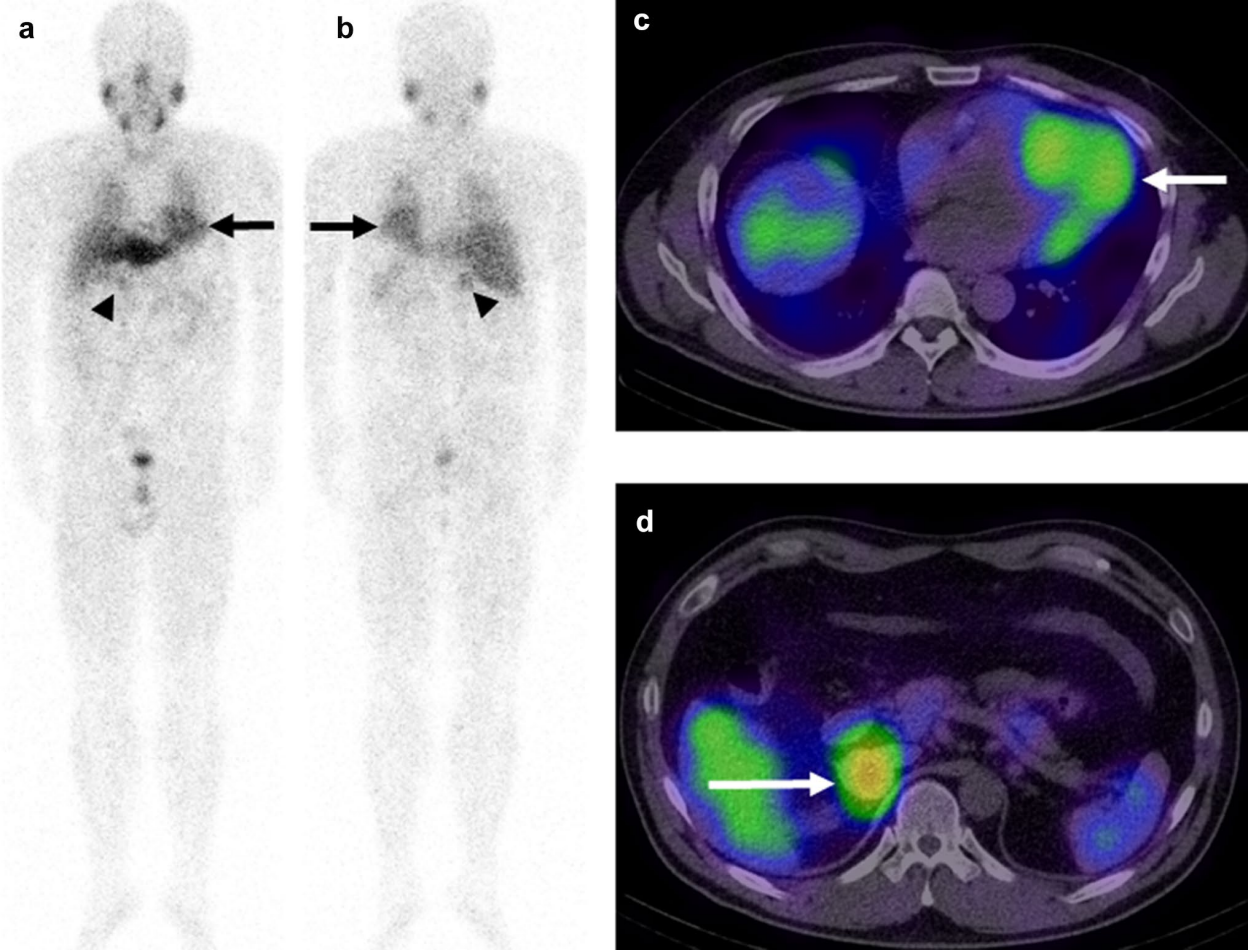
A 50-year-old male patient with right adrenal pheochromocytoma with normal cardiac function. The [^123^I]-MIBG anterior (a) and posterior (b) planar images show the normal cardiac uptake (arrow, score 2) and the pheochromocytoma uptake (arrowhead, score 2). The [^123^I]-MIBG SPECT/CT images show the [^123^I]-MIBG uptake in both the myocardium (c, arrow) and pheochromocytoma (d, arrow). The myocardium SUVmax, H/M ratio, and pheochromocytoma SUVmax were 4.54, 2.66, and 6.19, respectively. The LVEF, LAVI, E/A, septal e′, and septal E/e′ ratio were 74.7%, 23.2 mL/mm^2^, 0.97, 11.2 cm/s, and 6.2, respectively. These echocardiographic findings indicate normal cardiac function
